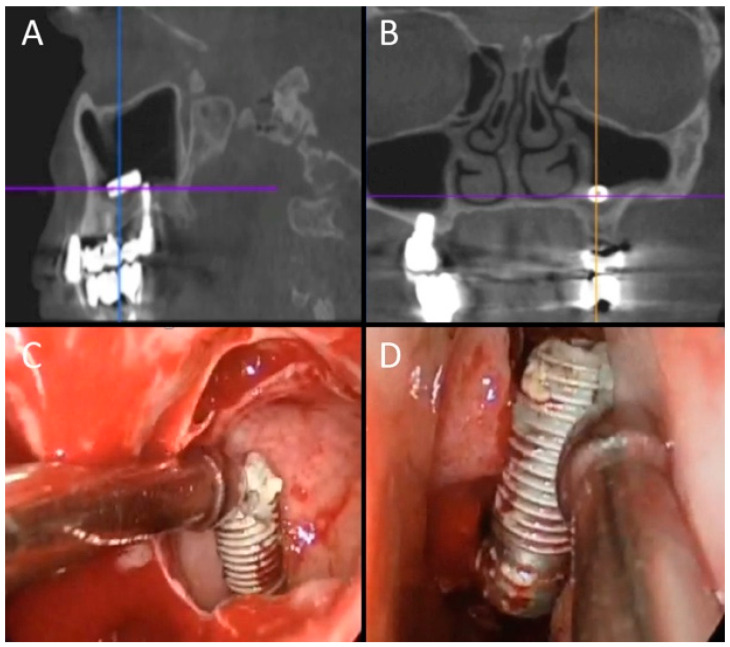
Female patient, 58 years old, who had undergone dental implant surgery several years earlier. CT performed for sinus pain documented migration into the left maxillary sinus ((A): sagittal scan; (B): coronal scan). An endoscopic surgical approach achieved removal of the foreign body ((C) and (D)).
